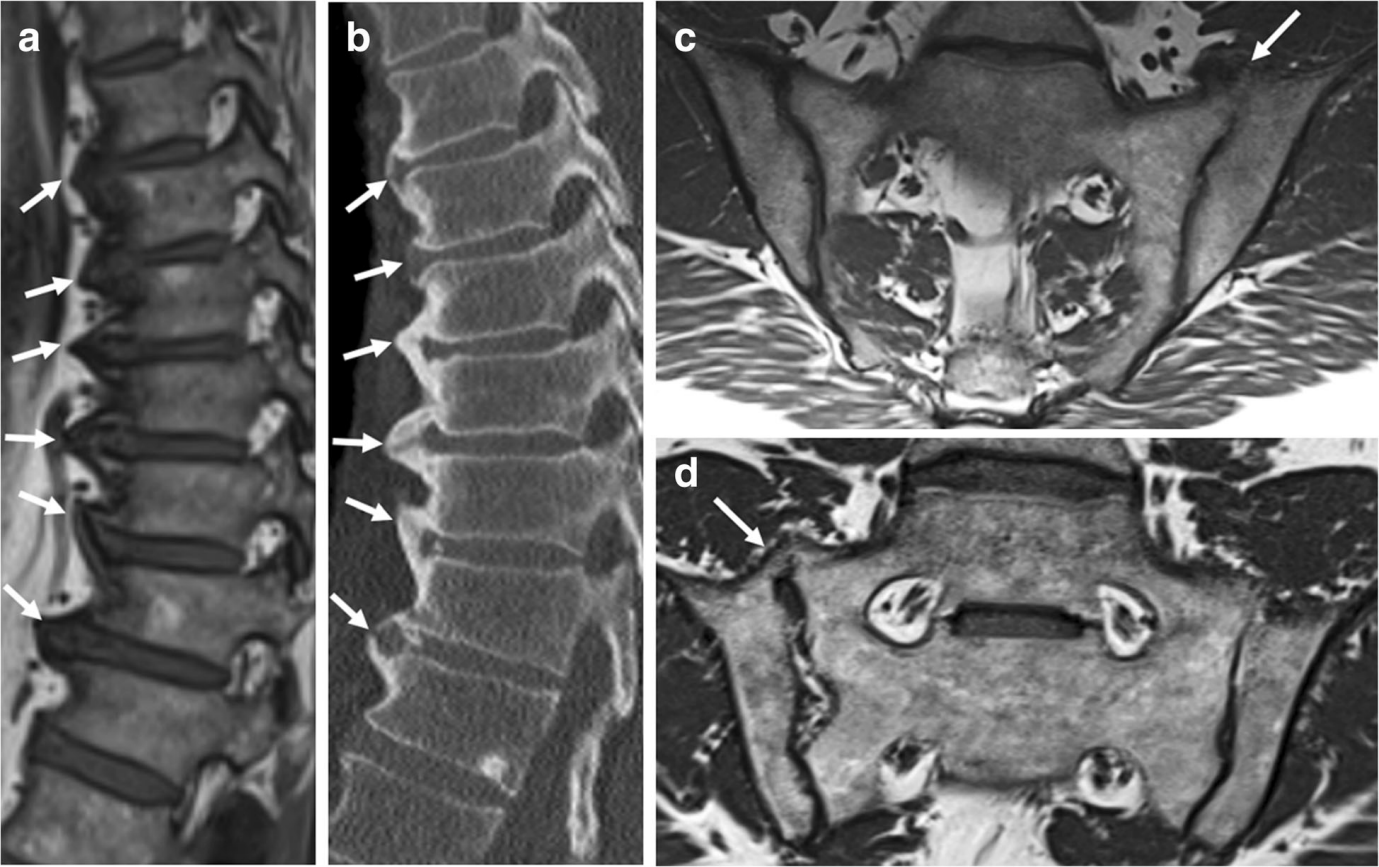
Pitfalls in imaging and diagnosis of new bone formation: DISH. a Sagittal T1-weighted MR image and (b) computed tomography (CT) show hyperproliferative ossification of the anterior longitudinal ligament, resulting in bulky horizontally oriented osteophytes (arrows) with—in this case—an average growth angle of > 45° from vertical. c, d Semicoronal T1-weighted MR images show bridging ossification (arrows) at the anterior and superior aspect of the sacroiliac joints
